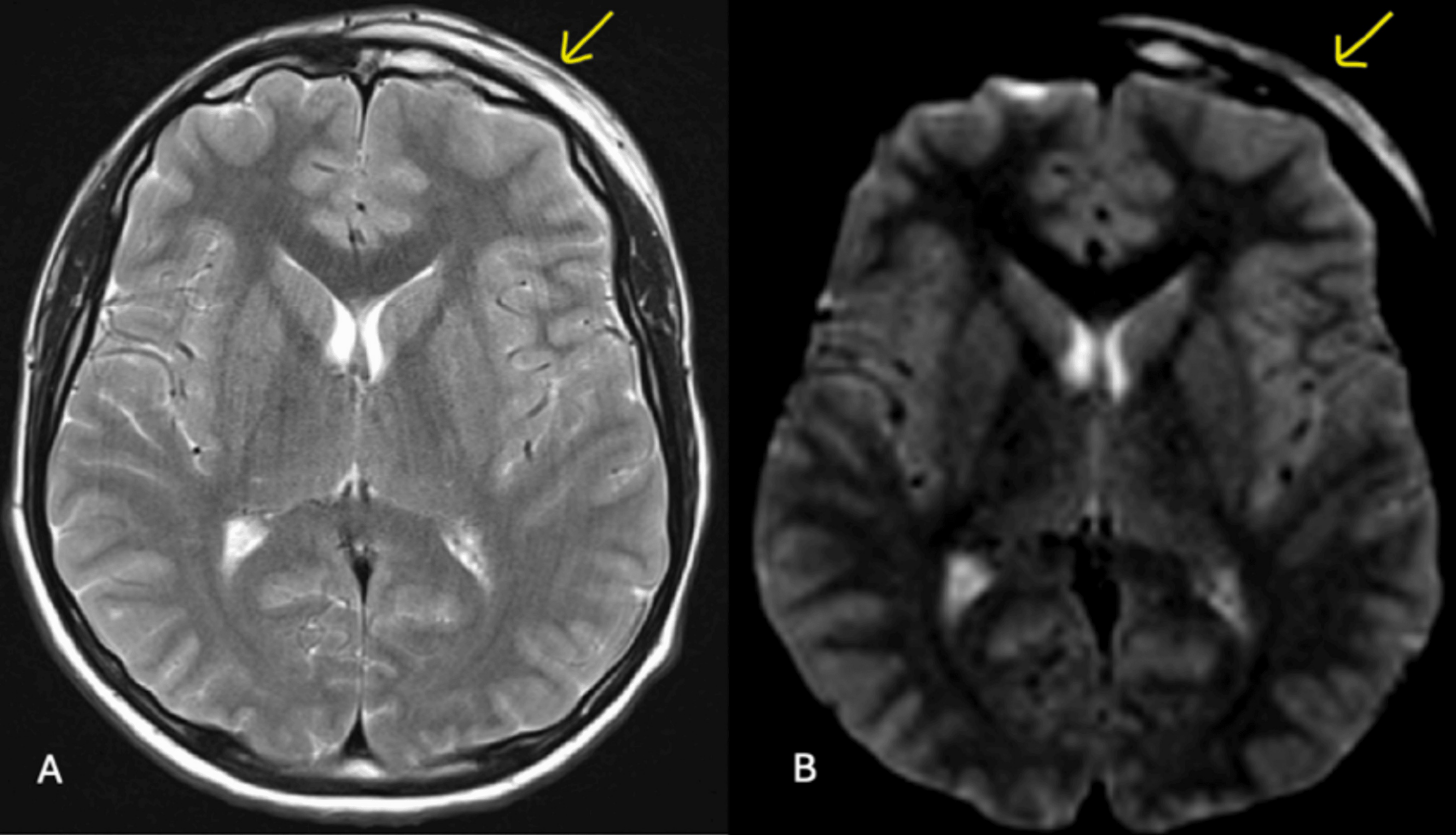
Non-contrast MRI head and sinus in the axial view shows left-sided subgaleal collection overlying the frontal bone in T2-weighted imaging (A) and diffusion-weighted imaging (B) (arrows).
